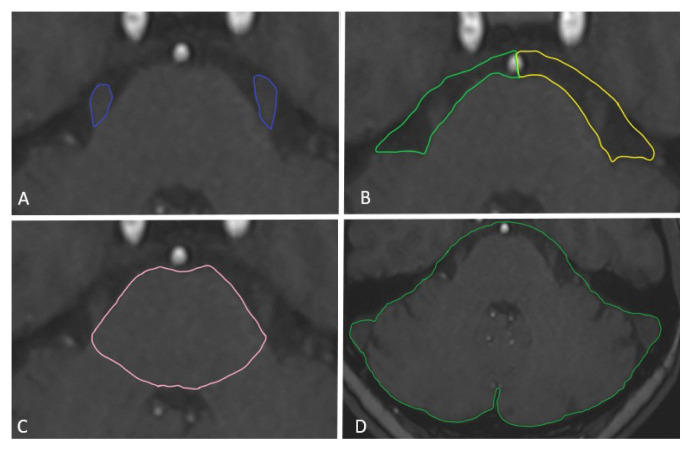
The figure shows the exemplary anatomical limit of the brain structures evaluated for volume measurements (cm^3^) on T1-weighted MRI scans in a 39-year-old female patient with TN. All volume measurements were automatically calculated on images transferred to a postprocessing Workstation (syngo.via version VB30A, Siemens Healthcare). (A); trigeminal nerve volume (blue area), (B); cerebellopontine cistern volume (yellow and green areas). (C); pons volume (pink area), (D); posterior fossa volume (green area).
